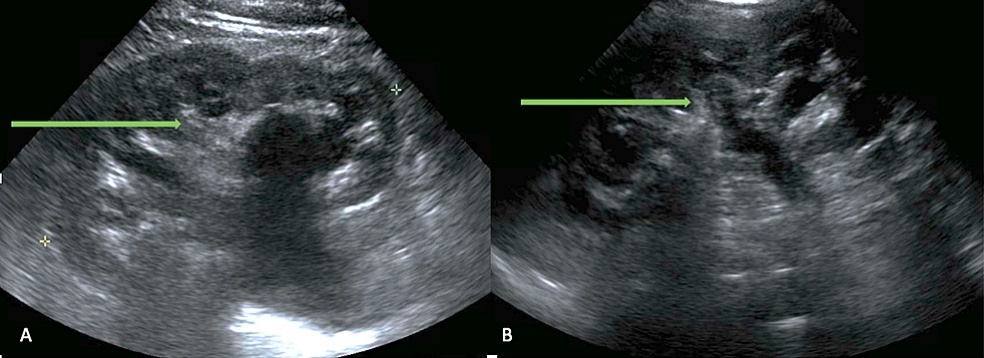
Ultrasound (sagittal view) of left kidney (A) and right kidney (B) showing improvement in hydronephrosis.
